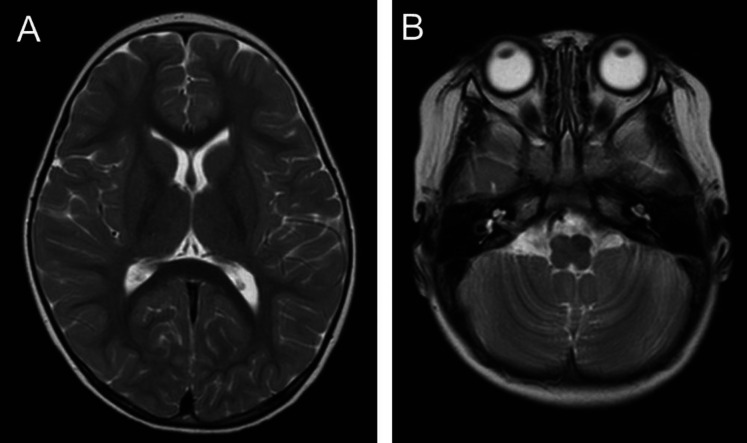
Brain MRI slices (A, cerebrum; B, cerebellum) acquired in the initial assessment did not show any abnormal findings
